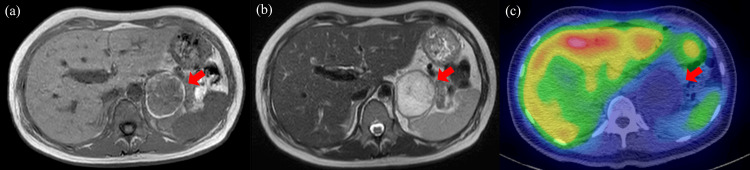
MRI and 123I-MIBG scintigraphy findings of the left adrenal mass (a) Simple sagittal T1-weighted MRI indicates a high signal area at tumor margins and a low signal area within the mass. (b) Contrast-enhanced MRI T2-weighted images show a low signal at the limbus and a high signal in the interior. (c) 123I-MIBG scintigraphy shows no accumulation at the mass.
